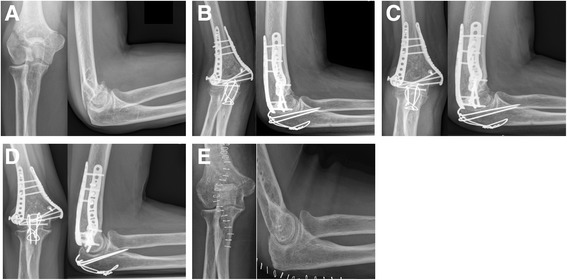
ORIF of a distal humerus fracture (AO 13-C3) with the Medartis® Aptus Elbow System. Radiographs of a 55-year-old female patient with a AO type 13-C3 fracture resulting from a bicycle accident: preoperative x-rays (a), postoperative results, 6 weeks (b), 12 weeks (c) and 6 months (d) following Medartis® Aptus Elbow plating. Implant removal could be obtained after fracture consolidation (e)
